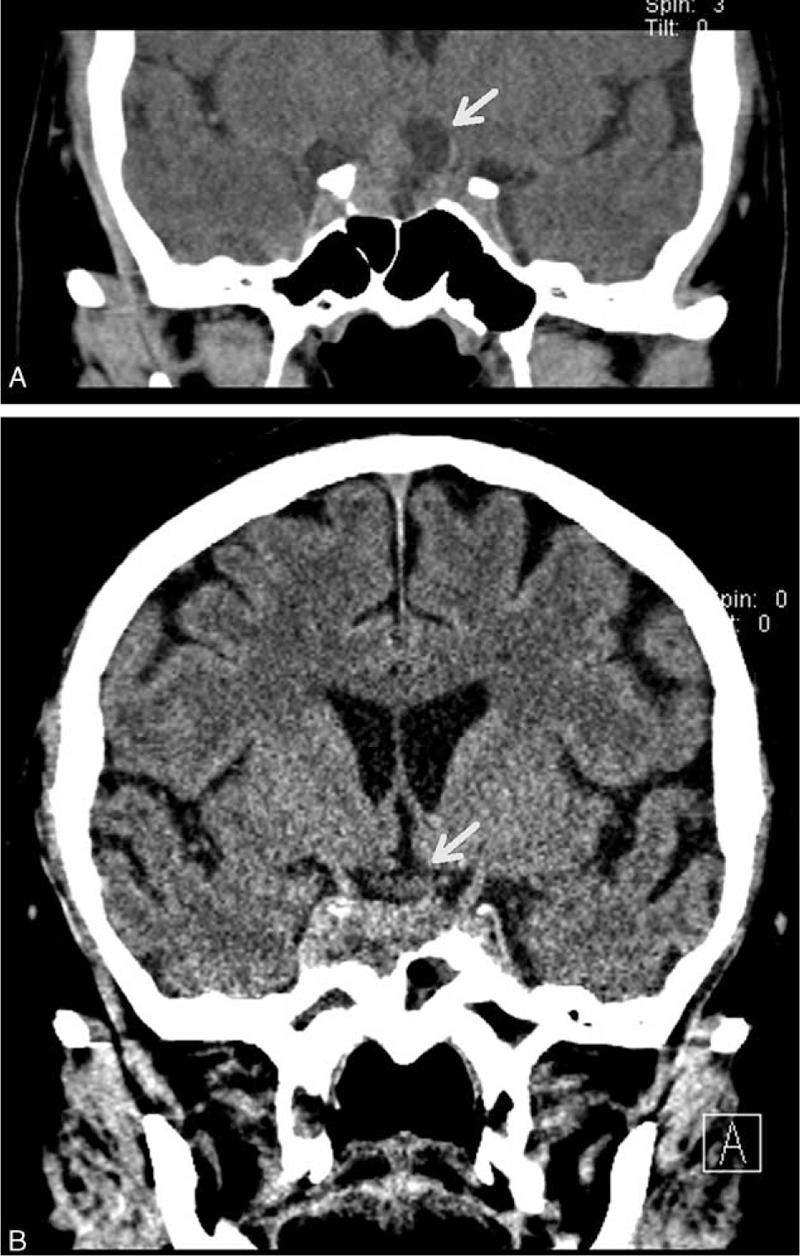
Head computed tomography (CT) scan: (A) before the 1st operation, coronal CT with multiple planar reconstruction shows a dilated sella turcica with a heterogeneously attenuated mass (white arrow); (B) at the 4th recurrence (October 2014), coronal CT multiplanar reconstruction shows the irregular suprasellar anatomy (white arrow) (likely a consequence of 3 previous operations and Gamma Knife radiotherapy).
